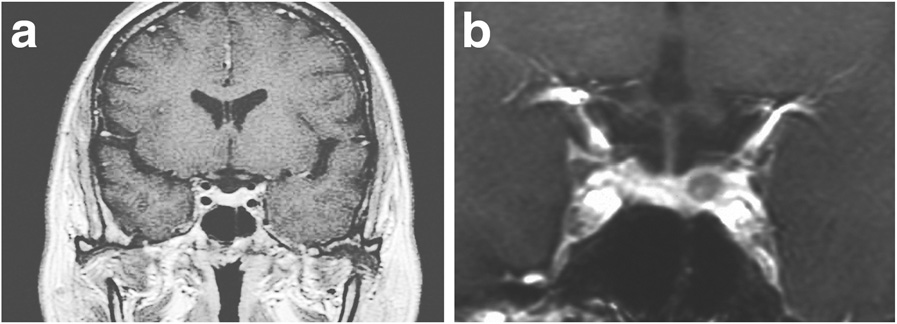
Initial coronal (a) magnetic resonance imaging showing normal appearance of the sella turcica and pituitary gland, and follow-up coronal (b) magnetic resonance imaging showing a less enhanced area in the left lateral wing of the sella turcica.
